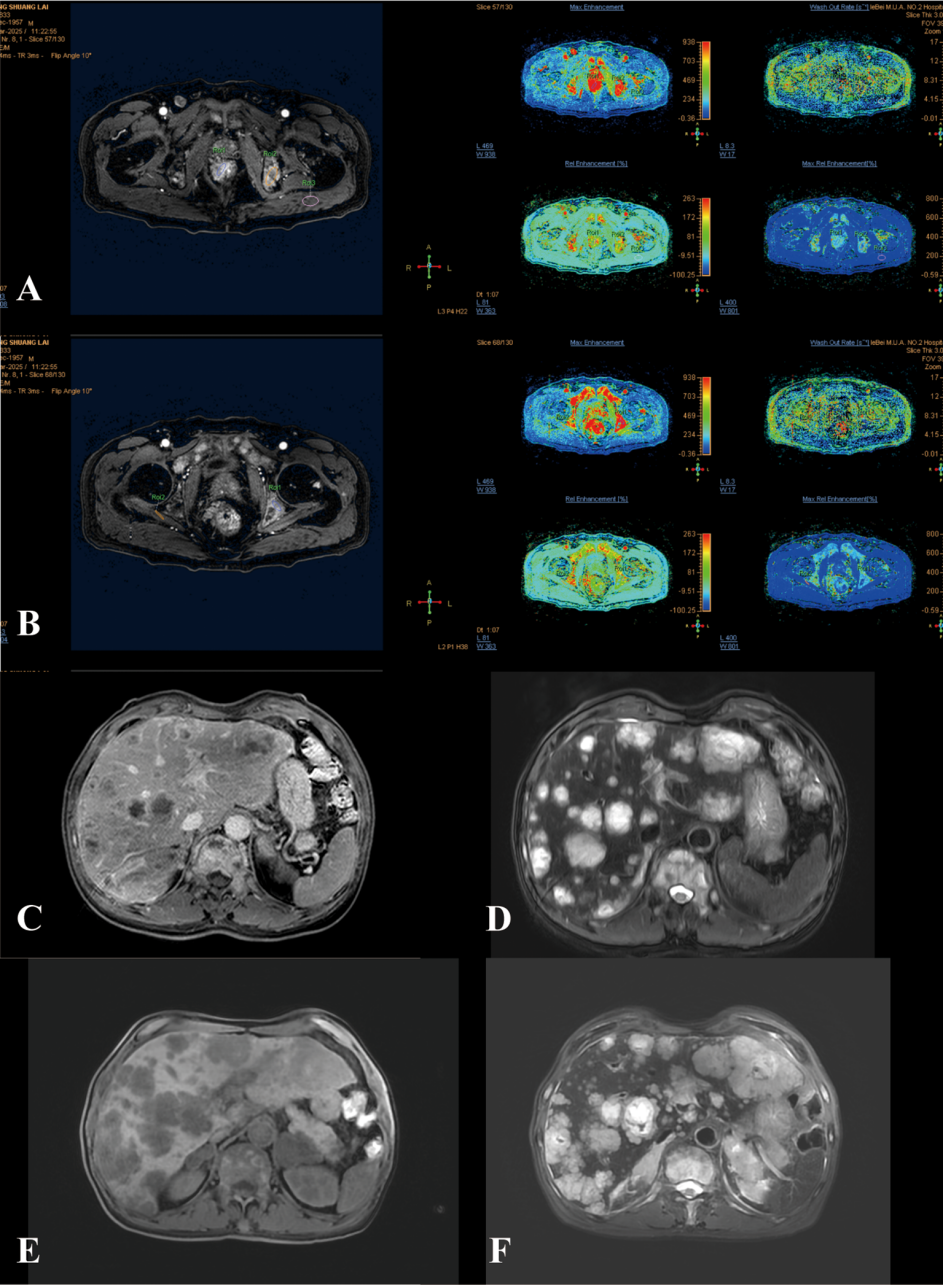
The changes in magnetic resonance (MR) during the patient’s treatment process. (A, B) The T1-weighted image of the rectum and the color T1 image of the metastatic lesion on MR at the time of hospital admission of the patient. (C, D) The T1 and T2 weighted magnetic resonance images of liver metastases during the stable period after radiotherapy and chemotherapy for the patient. (E, F) T1- and T2-weighted MR images of liver metastases in the terminal stage of the patient’s tumor.
